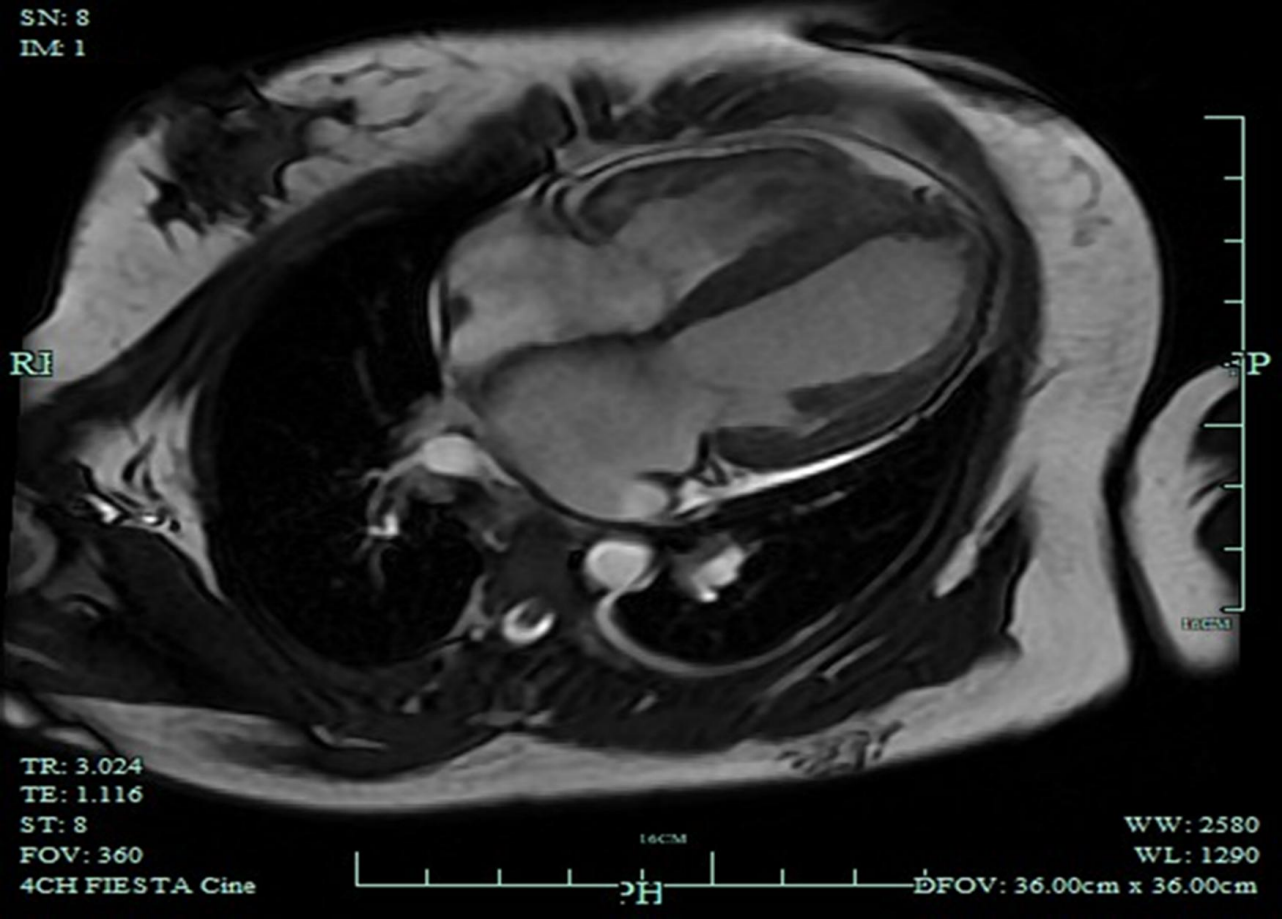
Multimodality cardiac imaging. Late gadolinium enhancement cardiac MRI displaying patchy mid-wall fibrosis with severely reduced systolic function (LVEF 21%).
